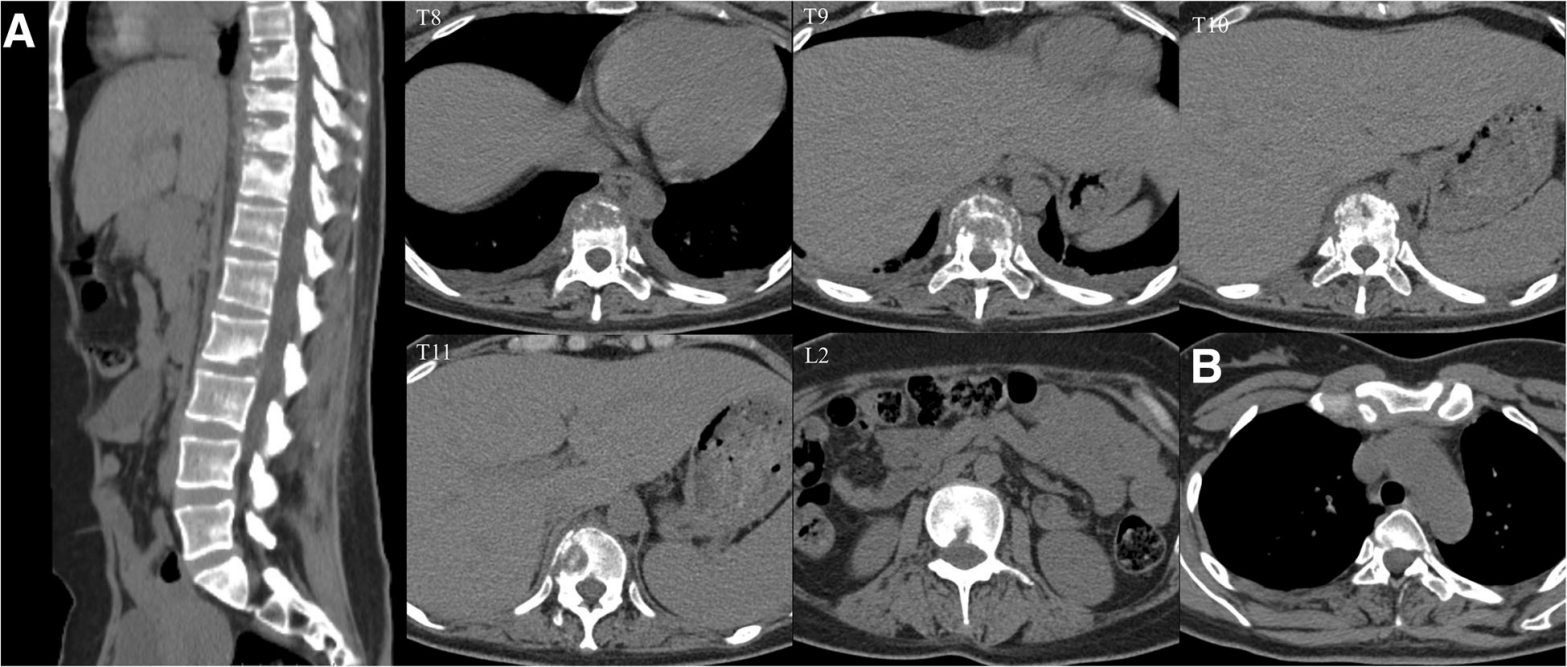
CT scans of the thoracic and lumbar spine revealed erosions, hyperostosis, and osteosclerosis in the T8–11 and L2 vertebral bodies and fractures in the T8–11 vertebral bodies (a). The cortical bone of the sternum and sternoclavicular joint was continuous without damage (b)
